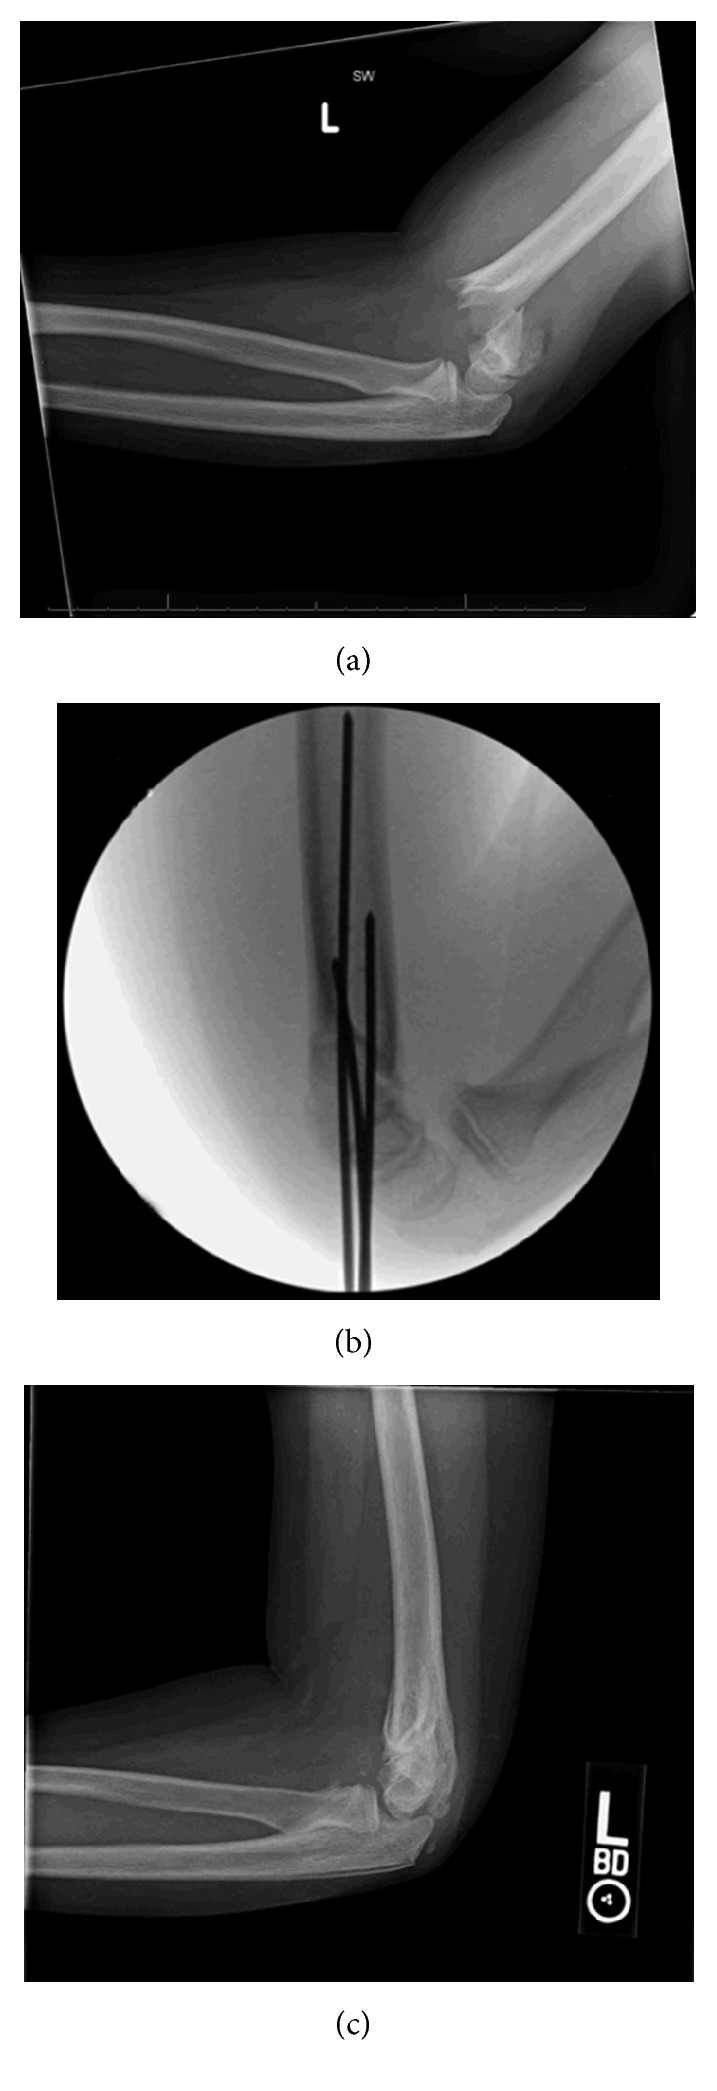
Plain films of the left elbow immediately after injury in the emergency department (a), intraoperative fluoroscopic film showing reduction and pin placement (b), and follow-up plain film imaging at 12 weeks after surgery (c).
